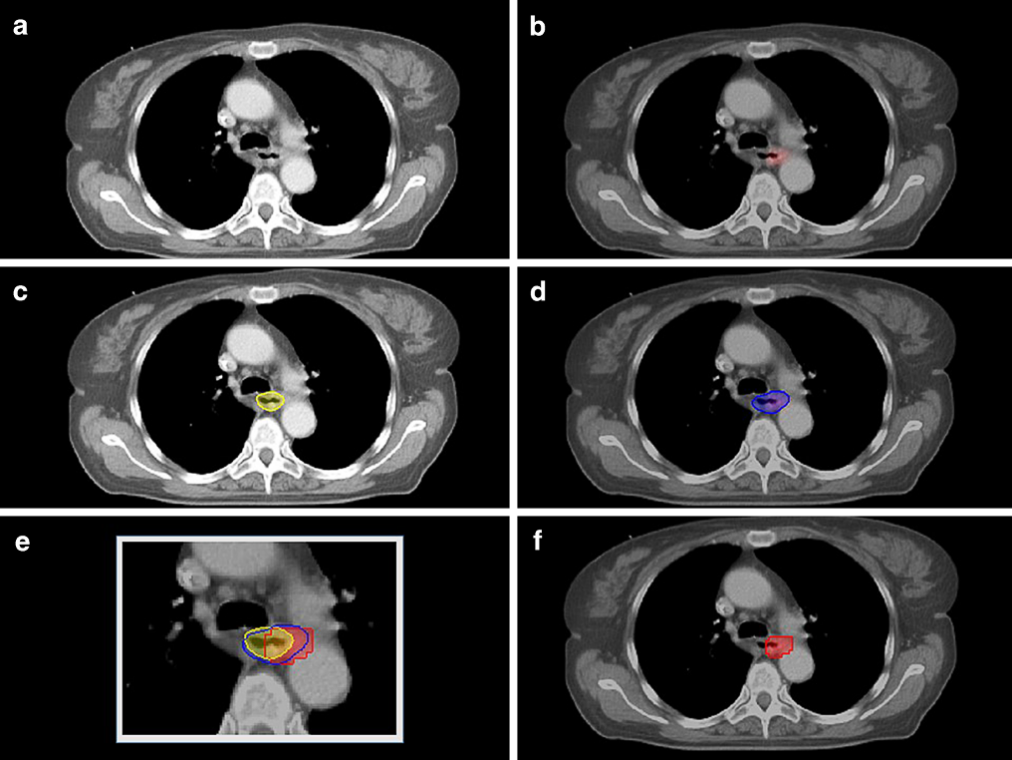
Manual gross tumor volume (GTV) delineation on a CT alone, b fused [F18] FDG-PET/CT, c GTV_CT_ (*yellow*), d GTV_PET/CT_ (*blue*), e matched GTV_CT_ (*yellow*), GTV_PET/CT_ (*blue*), and PERCIST-TLG (*red*), f PERCIST-TLG (*red*) semiautomated contour
